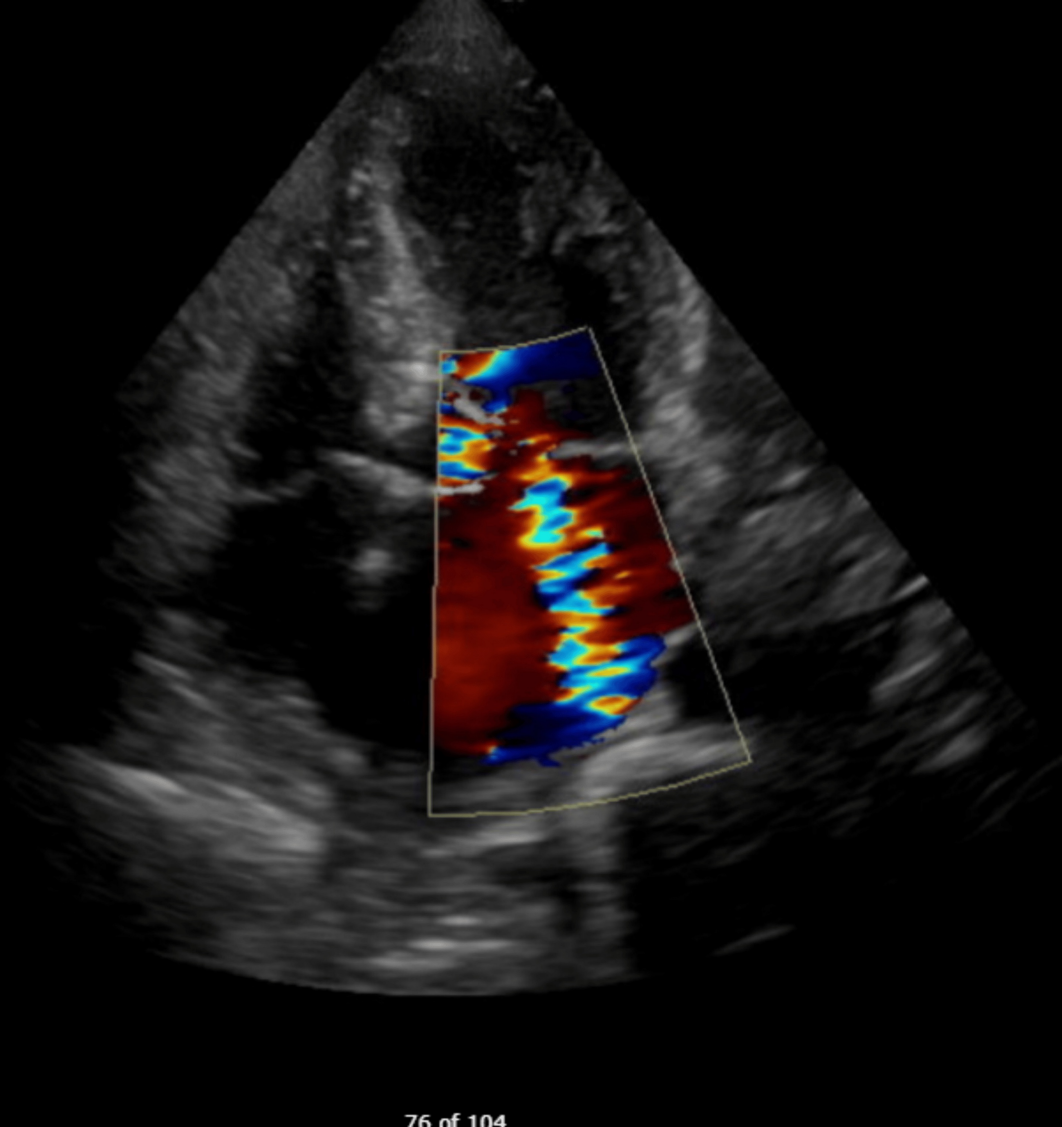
TTE showing regression of pericardial effusion. TTE: transthoracic echocardiogram.
